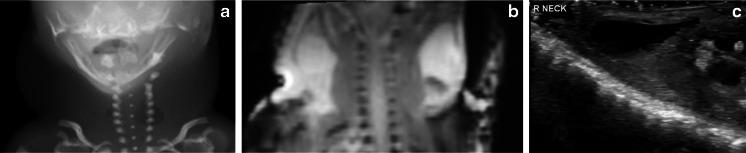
Cystic hygroma in female foetus of 14 weeks gestation. Hygroma was suspected on antenatal US scan (not shown), with the impression of a neck mass on postmortem radiograph (a). Postmortem MRI (b) was difficult to obtain because of size constraints, but postmortem US (c) was useful to delineate a bilateral cystic neck mass, which was confirmed to be cystic hygroma at autopsy
